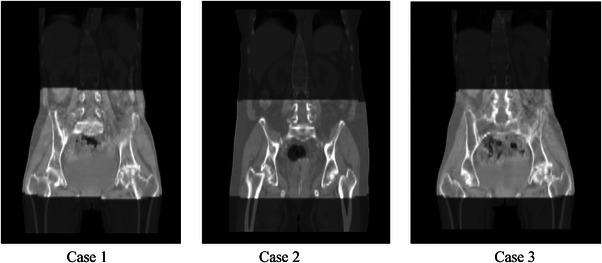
Display of rigid registration results for randomly selected three cases. The transparency is 0.5, the foreground image is CBCT and the background image is CT image. CBCT, cone‐beam computed tomography; CT, computed tomography.
